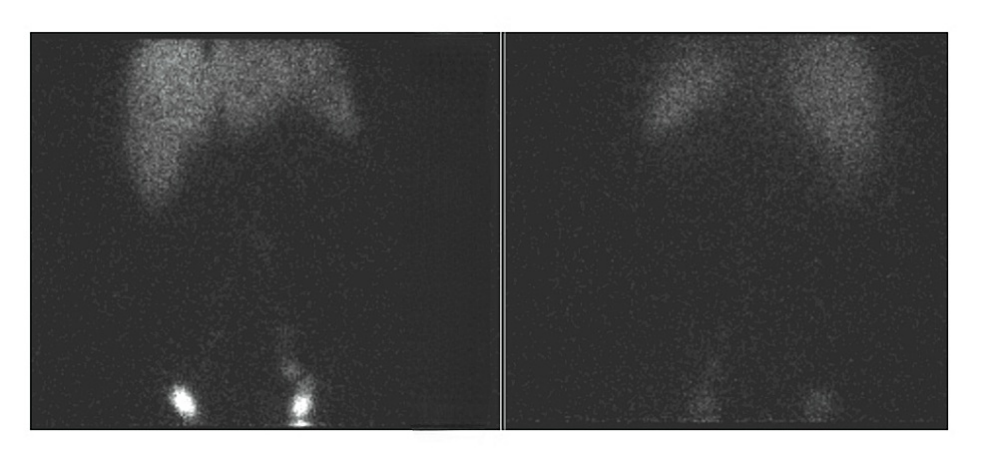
Tc-99m sulfur colloid scintigraphy The image shows the flow of radiotracer in the lymphatic channels after intradermal injection
